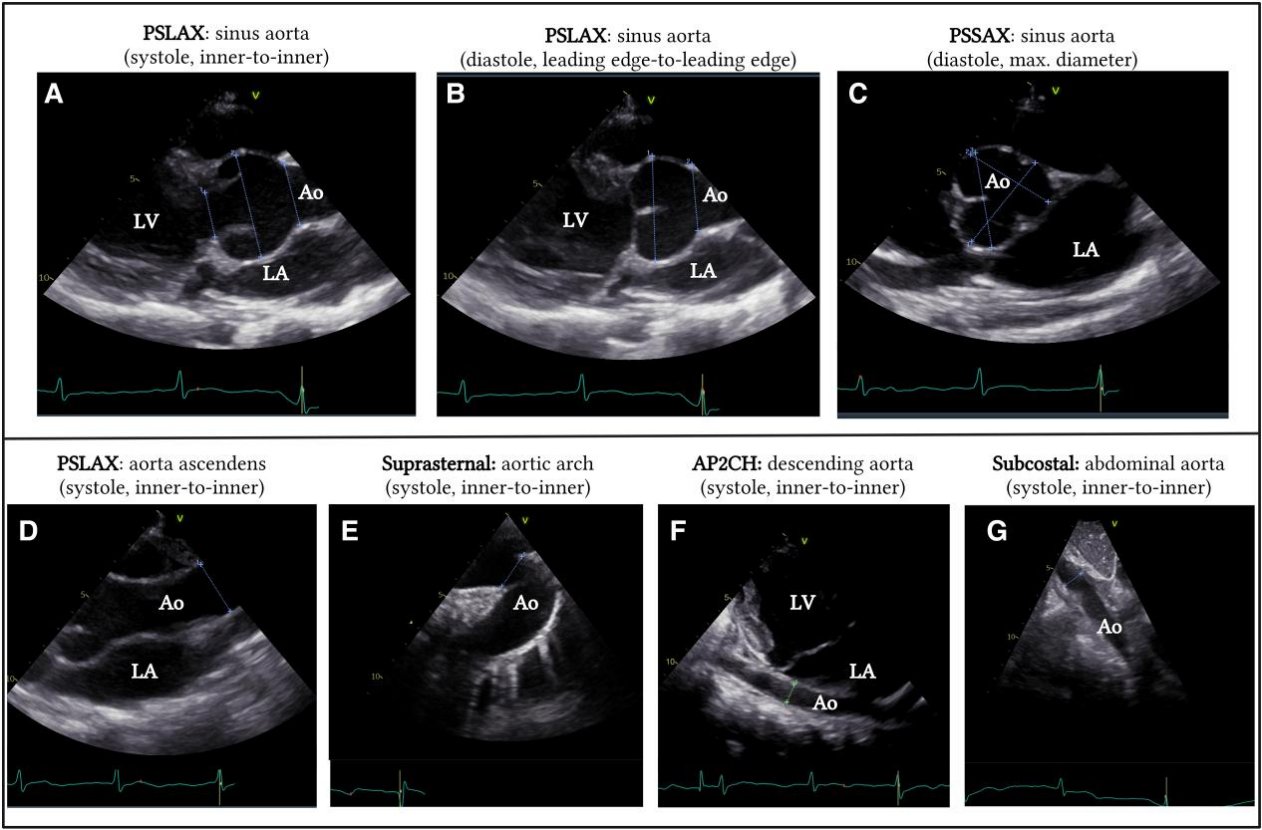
Echocardiographic imaging of the aorta at different levels. *A* Parasternal long-axis view of the aortic annulus, sinus of Valsalva, and sinotubular junction measured in systole using the inner-to-inner method. Measurements should be taken perpendicular to blood flow. *B* Parasternal long-axis view of the sinus of Valsalva and sinotubular junction measured in diastole using the leading edge-to-leading edge method. *C* Parasternal short-axis view of the aortic valve measured in diastole and using the largest aortic diameter. *D* Parasternal long-axis of the ascending aorta, measured in systole at the level of the right pulmonary artery, using the inner-to-inner method. *E* Suprasternal view of the aortic arch measured in systole between the truncus brachiocephalicus and the left carotid artery, using the inner-to-inner diameter. *F* Modified apical two-chamber view of the descending aorta measured at the level of the left atrium in systole using the inner-to-inner method. *G* Subcostal view of the abdominal aorta at the level of the liver, measured in systole using the inner-to-inner diameter. Ao, aorta; AP2CH, apical two-chamber view; LA, left atrium; LV, left ventricle; PSLAX, parasternal long-axis view; PSSAX, parastenal short-axis view
